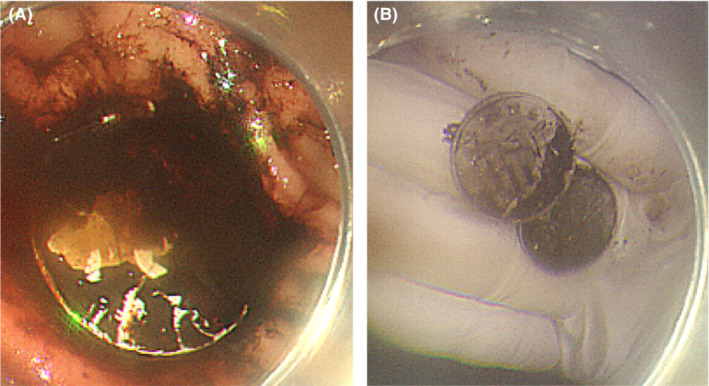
(A) Coins were embedded in the stomach body. (B) Two coins were removed endoscopically, although they appeared to be a single coin on X‐ray and CT.
